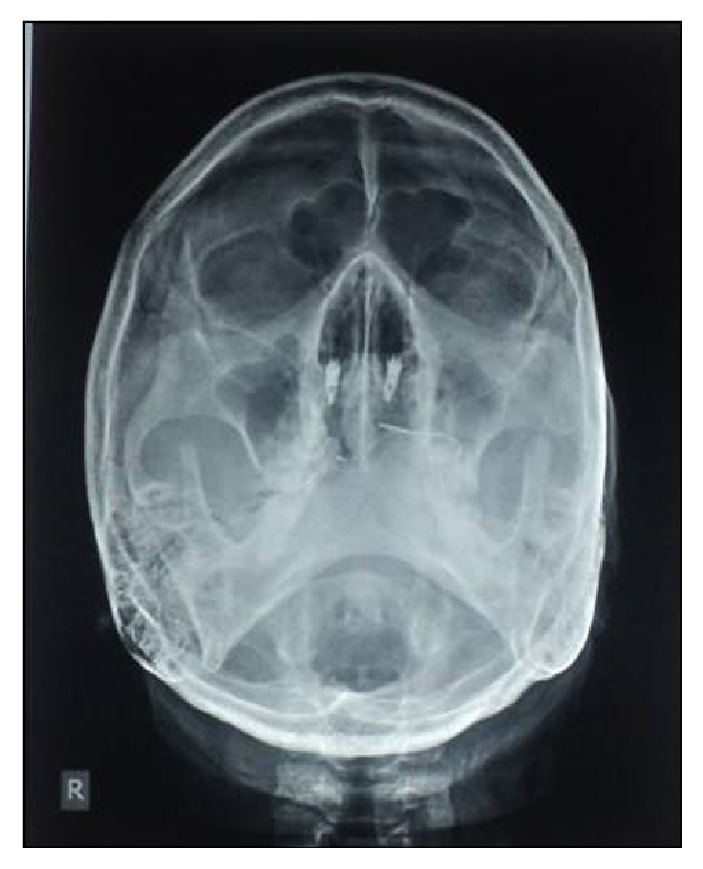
Postoperative PNS water's view radiograph showing implant in position.
